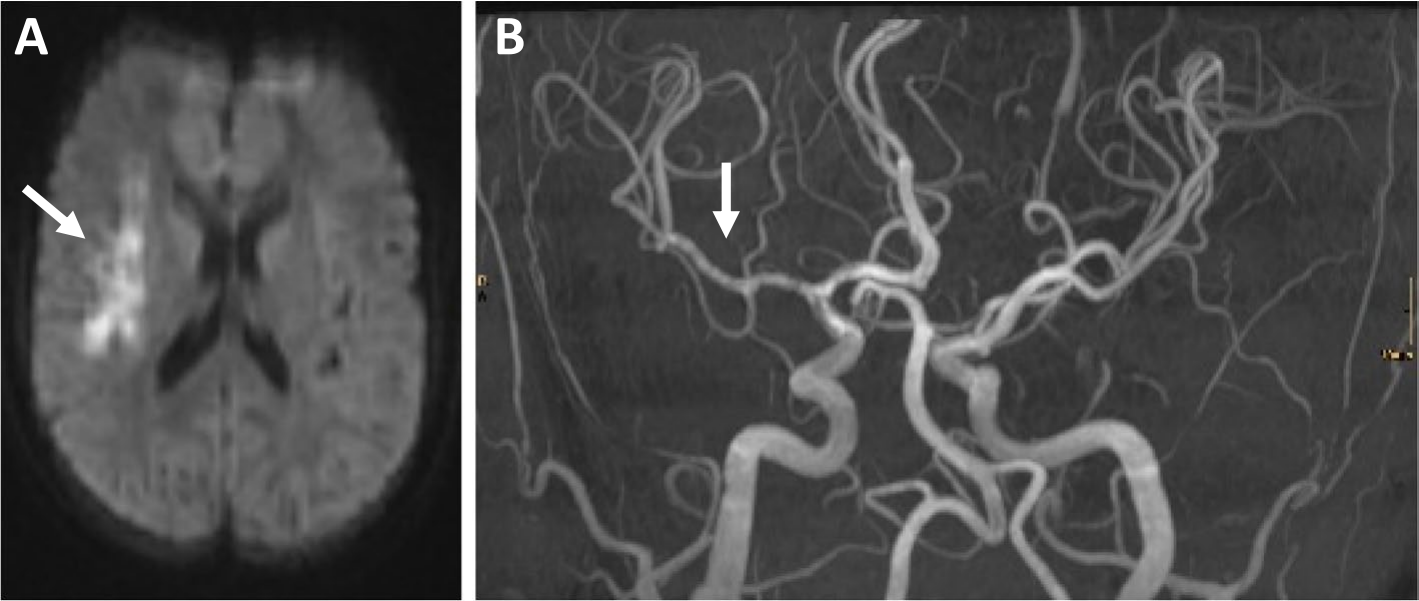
Cerebrovascular involvement in jSLE. A Infarction in a 15-year-old patient. Transversal MRI with diffusion disorder in right temporal lobe; DWI sequences. B Vasculitis of the *arteria cerebri media* with vascular irregularities in MR angiography (MRA)
